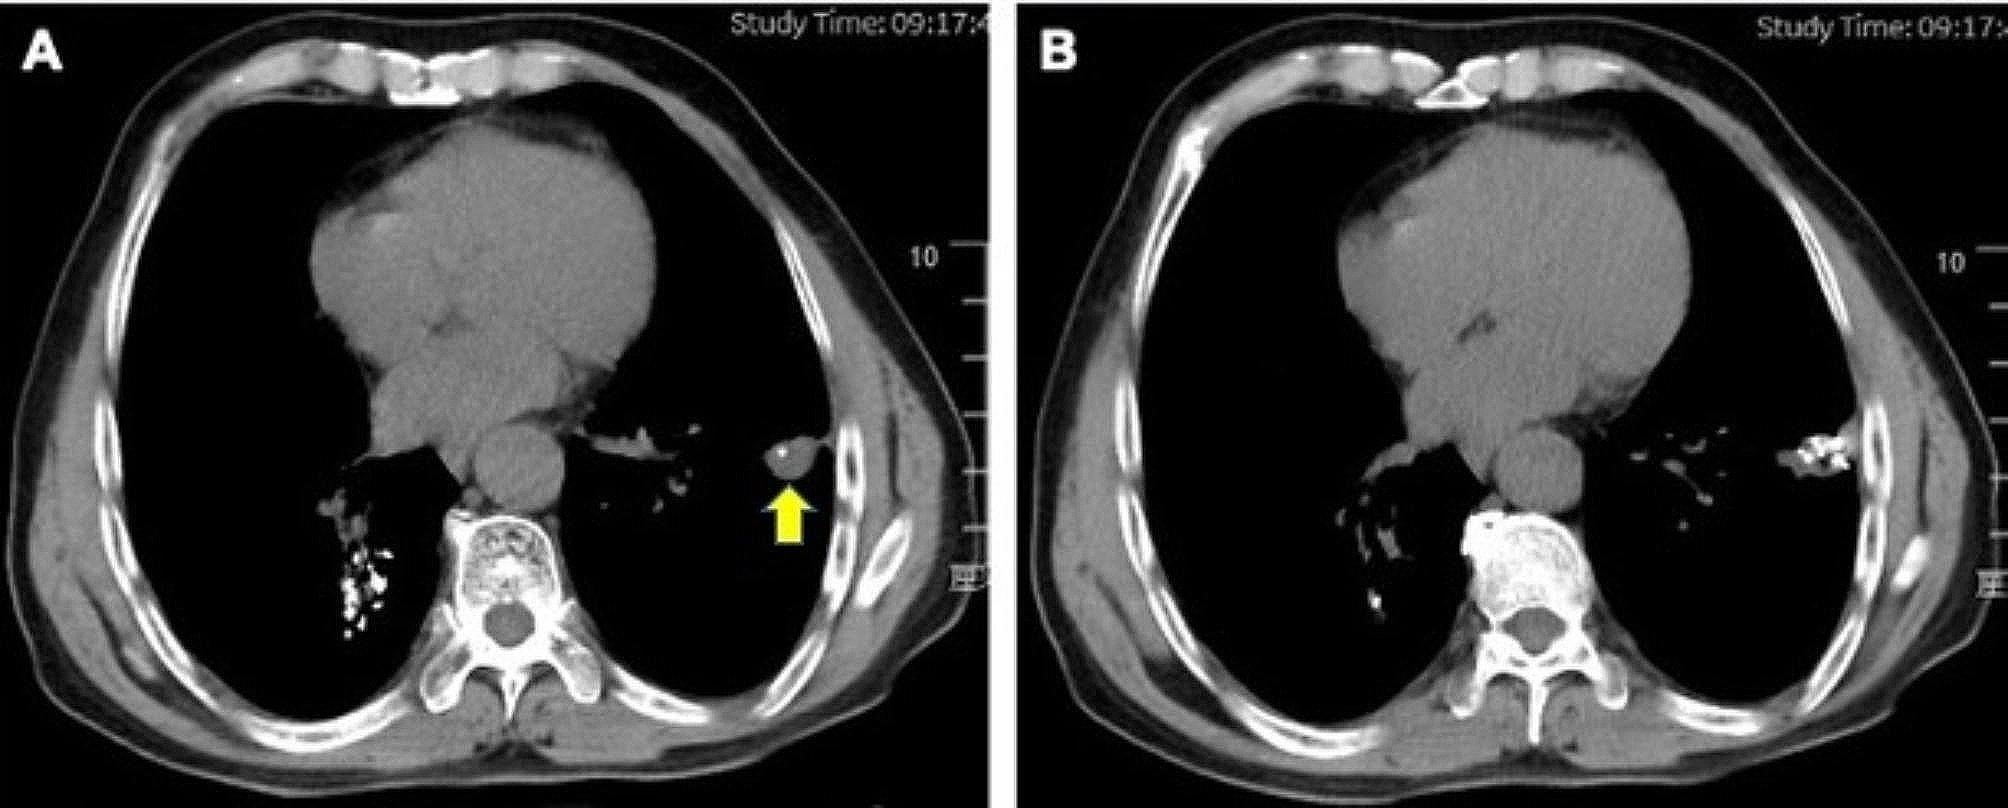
Another representative case of L-SABT. Follow-up CT at 5 months indicated local tumor progression (yellow arrow)
